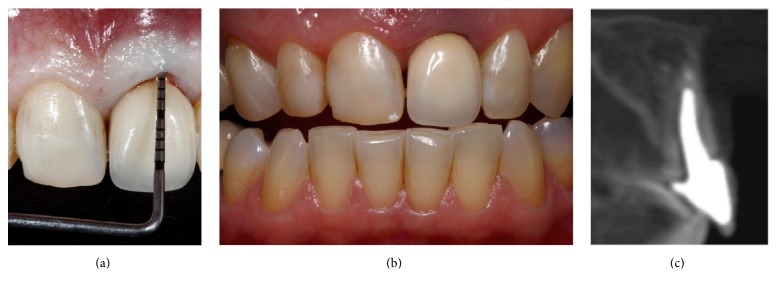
An oblique fracture occurred in tooth 21 due to occlusal trauma. Clinically the patient presented a deep periodontal pocket in tooth 21 (a) thin biotype and shorter clinical crowns in teeth 13 and 12 (b). The buccal bone wall loss and periapical lesion were observed in the CBCT (c).
